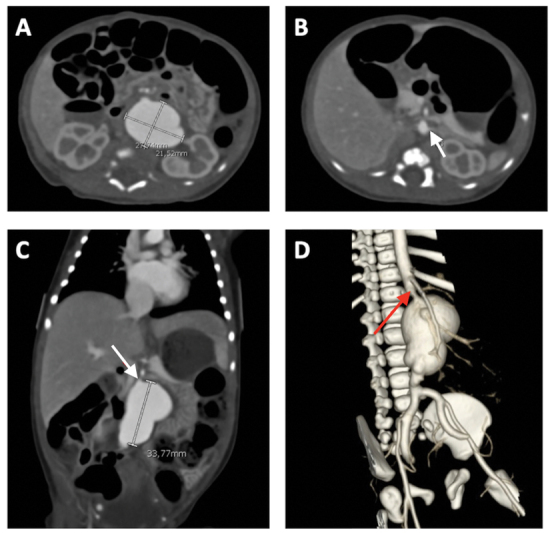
Initial computed tomography angiogram of chest, abdomen, and pelvis. Images (
